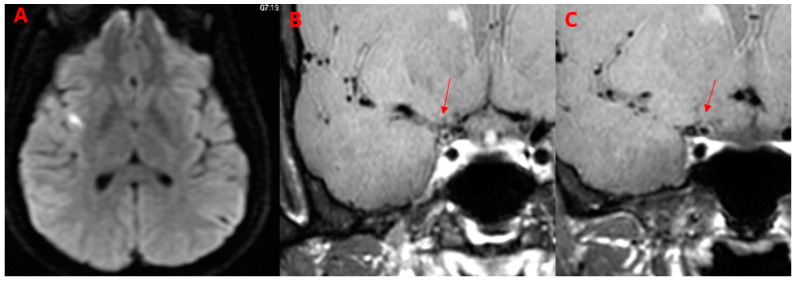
Patient (M/32) with acute onset of headache, dysarthria, and left arm weakness. The diagnosis was PCNSV. (A) Axial DWI image shows a right insular hyperintense area with restricted diffusion. (B,C) Coronal BB T1w VWI after administration of the contrast agent shows an intense, concentric wall enhancement (arrows) of the right sovraclinoideal internal carotid.
